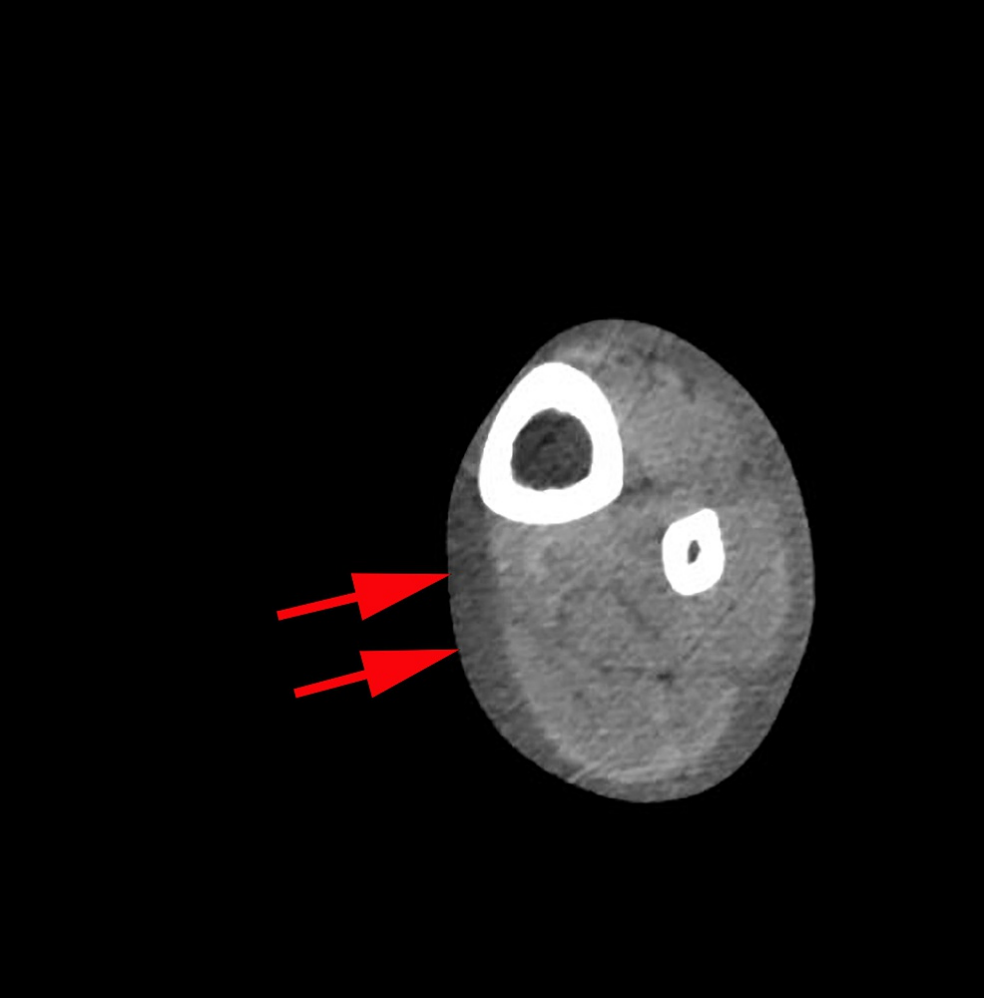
CT scan of the left lower extremity. CT of the left lower extremity revealed diffuse subcutaneous edema with fat stranding (red arrows), suggestive of cellulitis, but was negative for necrotizing fasciitis or osteomyelitis.
